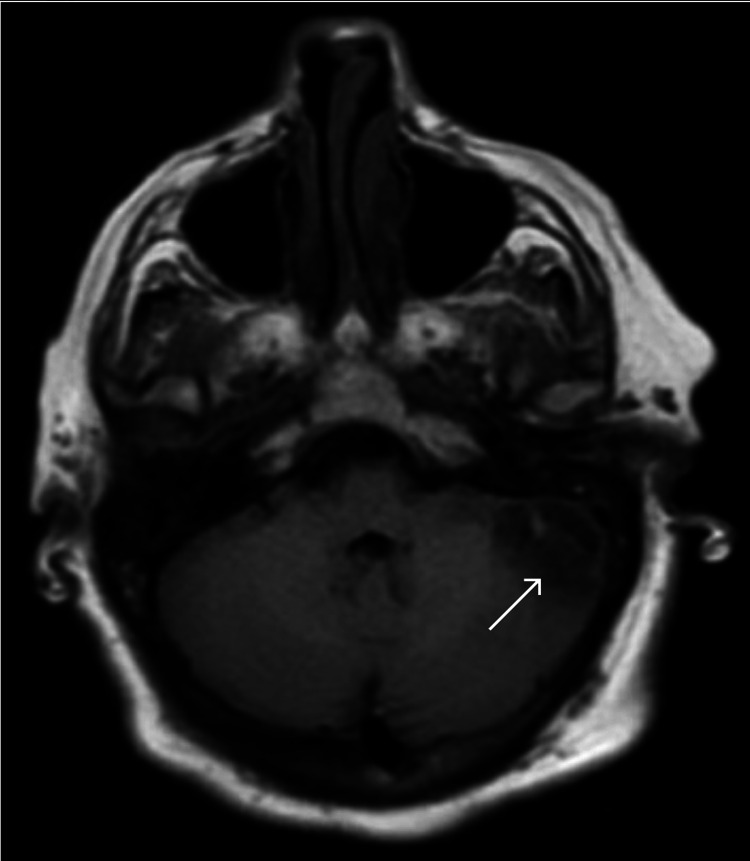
MRI brain showing left cerebellitis and posterior fossa epidural abscess on T1 sequence MRI: Magnetic resonance imaging
